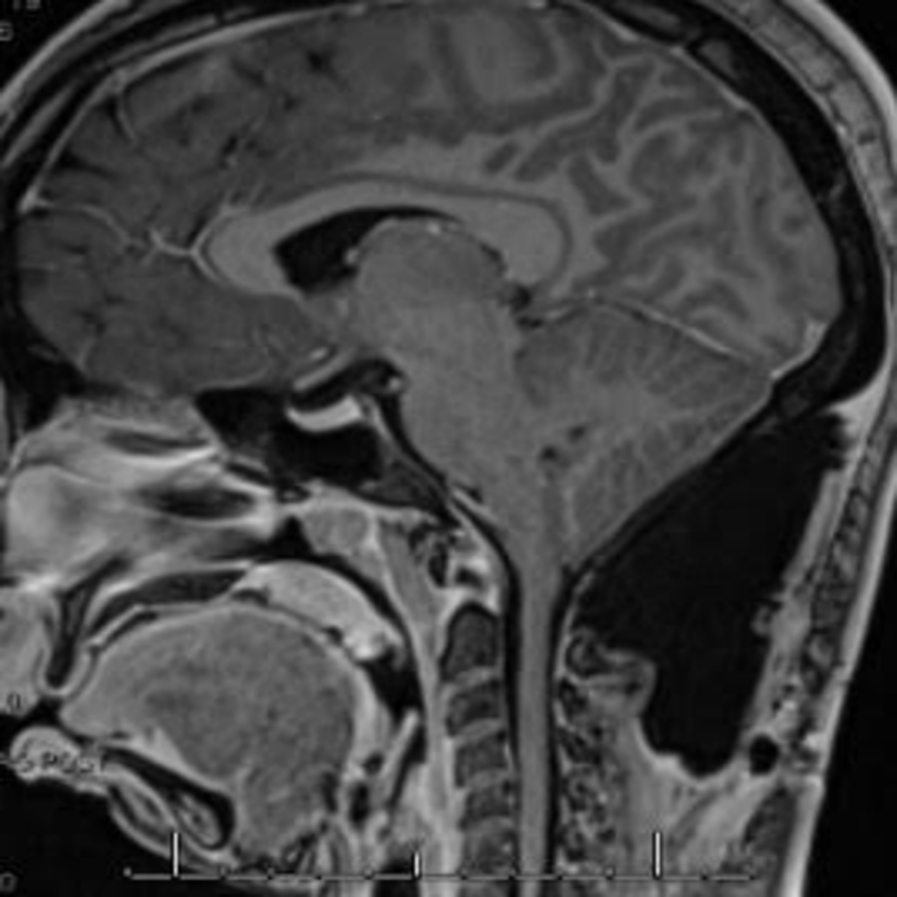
Postoperative Sagittal MRI After a Suboccipital Craniectomy in a Patient with a Delayed Diagnosis of Idiopathic Intracranial Hypertension
